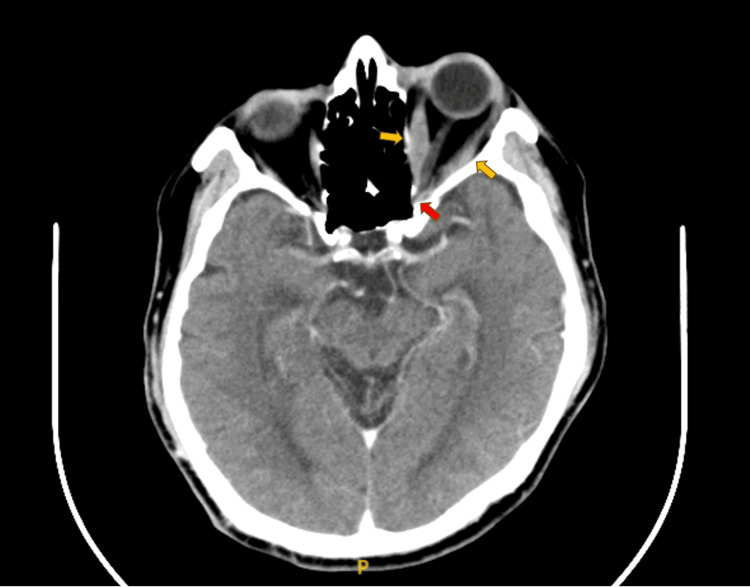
Axial contrast-enhanced CT (CECT) of the orbits showing tendon-sparing enlargement of the left medial and lateral rectus muscles (yellow arrows) with apical crowding and compression of the optic nerve (red arrow). Image obtained at the Radiology Department, Hospital Shah Alam, Malaysia.
